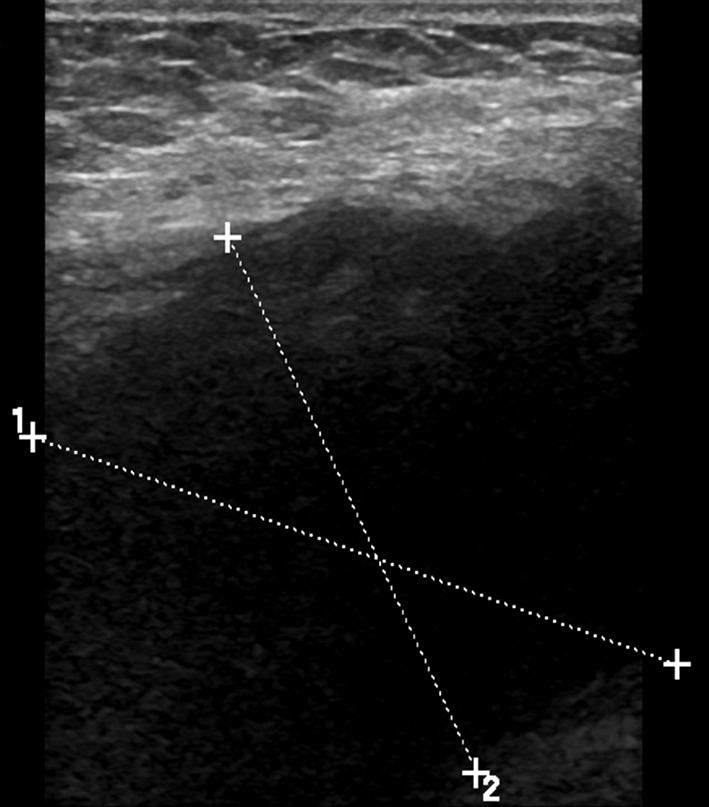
Ultrasound right axilla shows a poorly defined hypoechoic mass (callipers).
